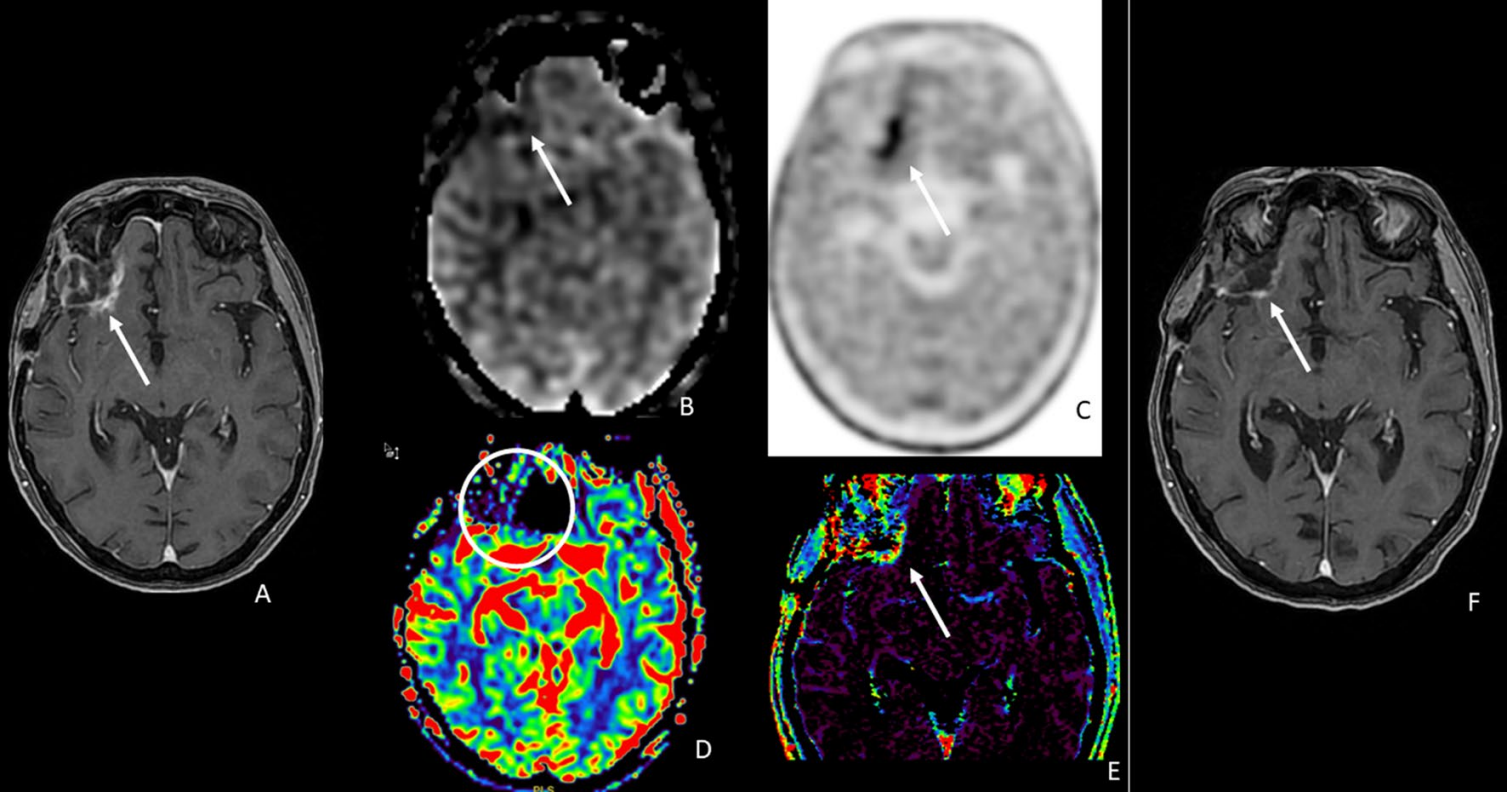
A representative case of high-grade glioma with a linear enhancing lesion in the left front-basal region, close to the surgical site, occurred 8 months after radiation therapy (a, arrow). The enhancing lesion did not show an increase in ASL_CBF (b, arrow); DSC-rCBV evaluation was difficult because of the proximity to bone structures (d, circle); and DCE_Ve was slightly increased (e, arrow). The uptake of 18F-DOPA was high (c, arrow). Six months of MRI follow-up showed a massive reduction in contrast enhancement (f, arrow). ASL correctly classified the lesion, whereas the linear 18F-DOPA uptake close to the surgical site could be the expression of macrophages activation. ASL = arterial spin labeling; CBF = cerebral blood flow; DSC = dynamic susceptibility contrast enhanced perfusion; DCE = dynamic contrast enhanced perfusion; CBV = cerebral blood volume; Ve = fractional volume of the extravascular extracellular space
